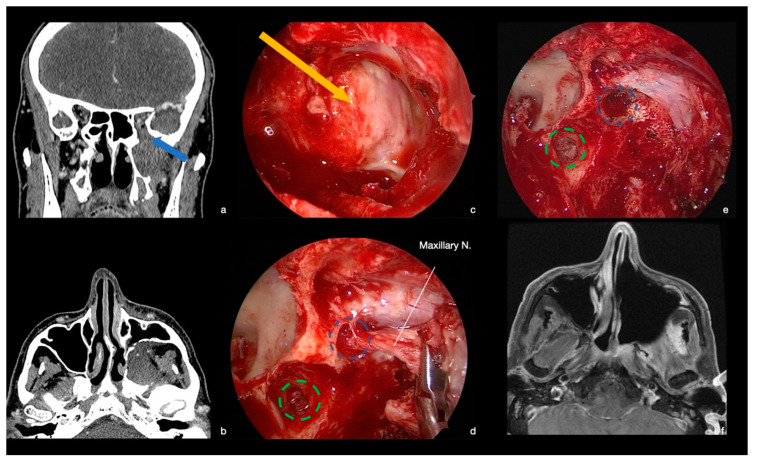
CT scan, MRI, and intra-operative endoscopic images (0° scope). (a,b) Coronal and axial NECT showing the PPF mass. An enlarged foramen rotundum, due to tumor infiltration of the maxillary nerve was observed (blue arrow). (c) Intra-operative image. After medial maxillectomy, at the posterior wall of the maxillary sinus, it was possible to observe a bulge directed anteriorly due to the presence of the mass in the PPF (yellow arrow). The infra-orbital nerve was evident in the roof of the maxillary sinus. (d) Intra-operative image. The maxillary nerve resulted infiltrated by the tumor and was resected up the foramen rotundum (blue circle), while the vidian nerve had been already resected at the opening of pterygoid canal (green circle). (e) Intra-operative image. At the end of tumor removal, no tumor remnants were observed and foramen rotundum (blue circle) and opening of pterygoid canal (green circle) are visible. (f) MRI. Axial slice. T1 w-sequence with contrast agent. Radical tumor resection with no surgical complications was confirmed.
